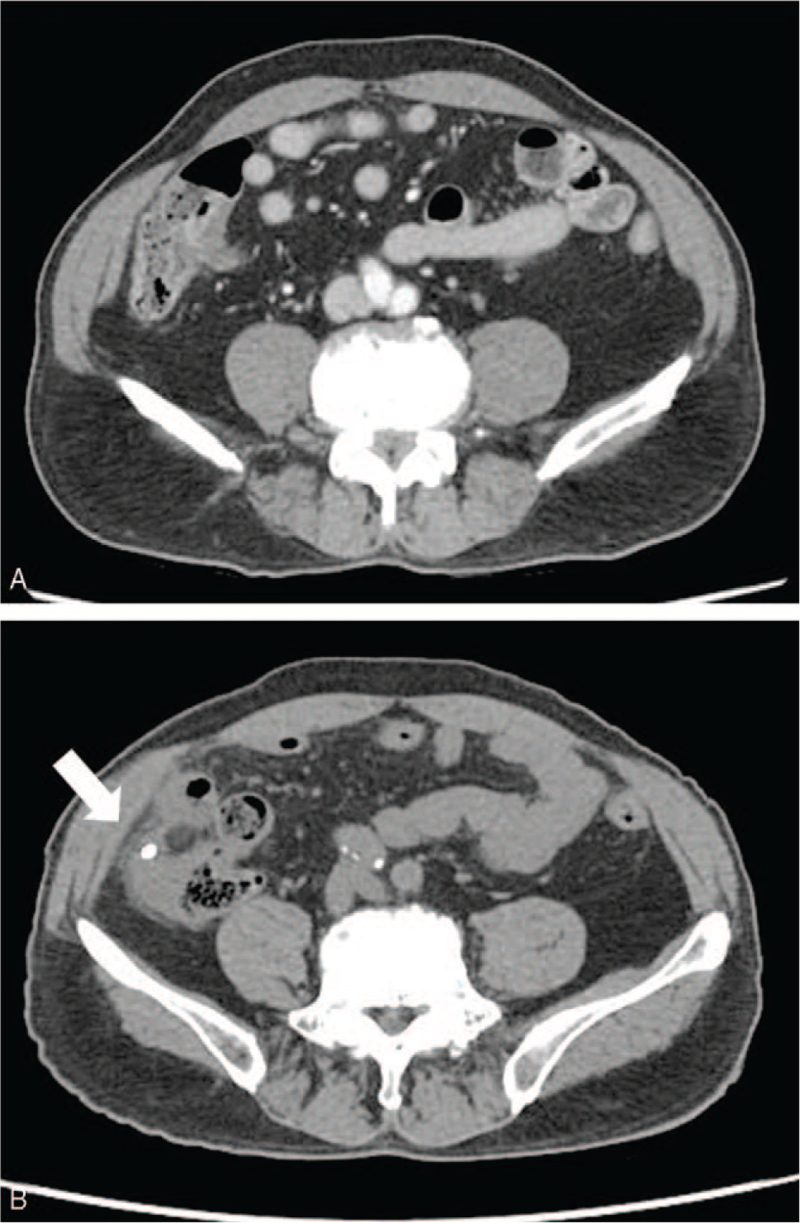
Abdominal computed tomography (CT) shows perforation of the proximal ascending colon with localized peritonitis by a migrated biliary stent.
